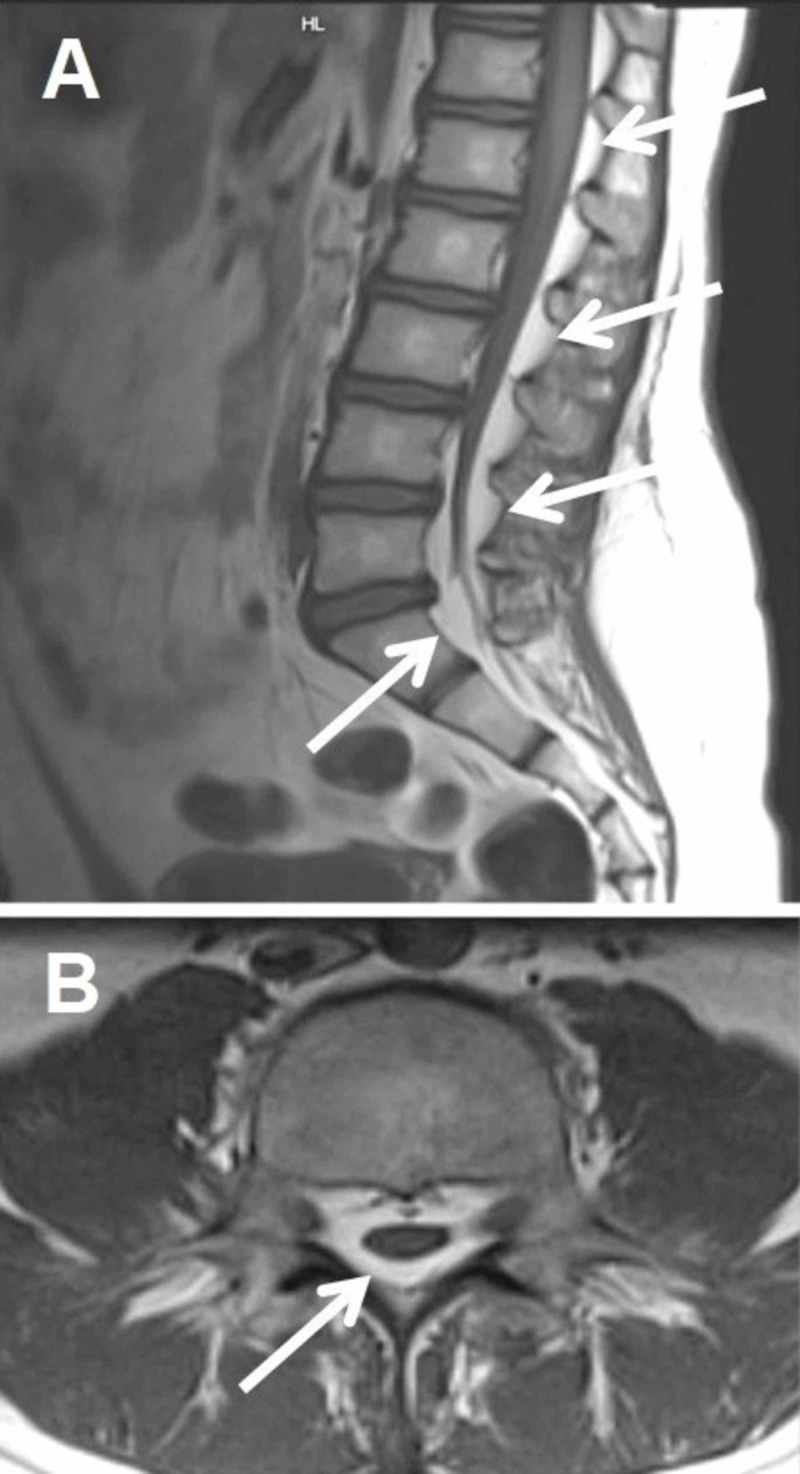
A sample MRI of the lumbar spine, demonstrating spinal epidural lipomatosis The arrows point to selected areas of the pathological accumulation of fatty tissue in the epidural space. T1 weighted images: A, sagittal plane; B, axial plane.
